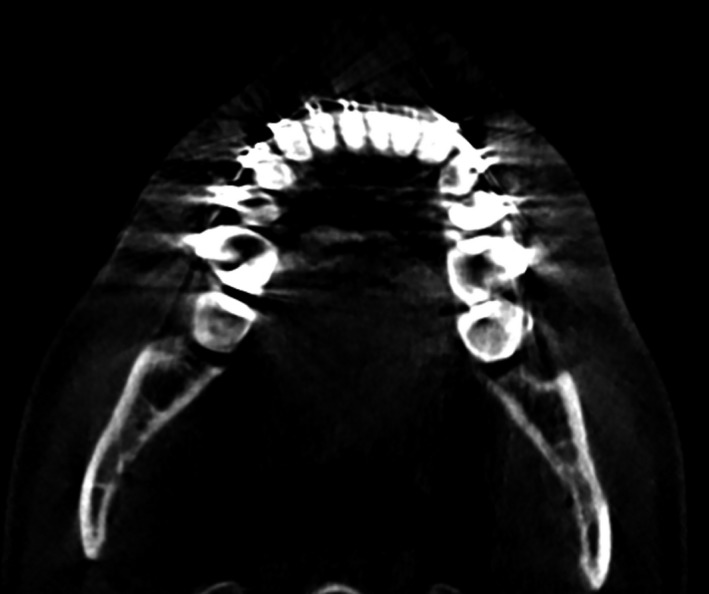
Example of metal artifacts in a cone‐beam computed tomography image of the mandible.
